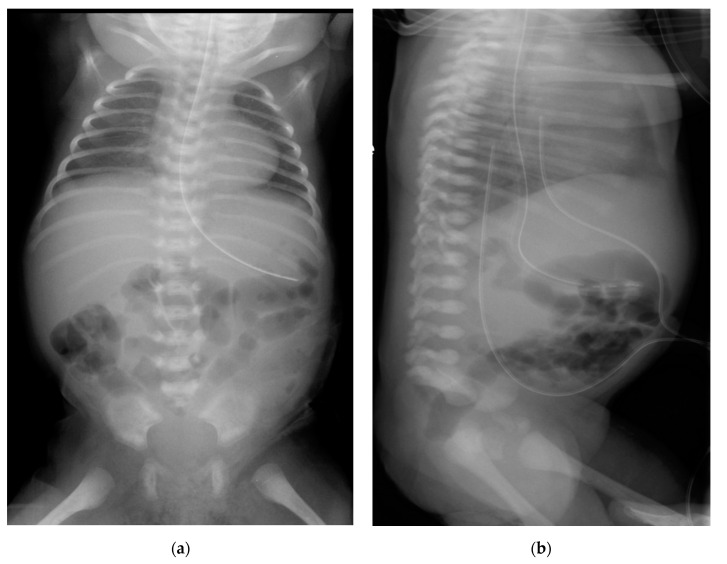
Plain (anteroposterior (a) and lateral (b)) radiograph of chest and abdomen showing absent sacrum. Both the umbilical arterial and venous catheters on the lateral view were not appropriately positioned but was pulled back to optimal site.
